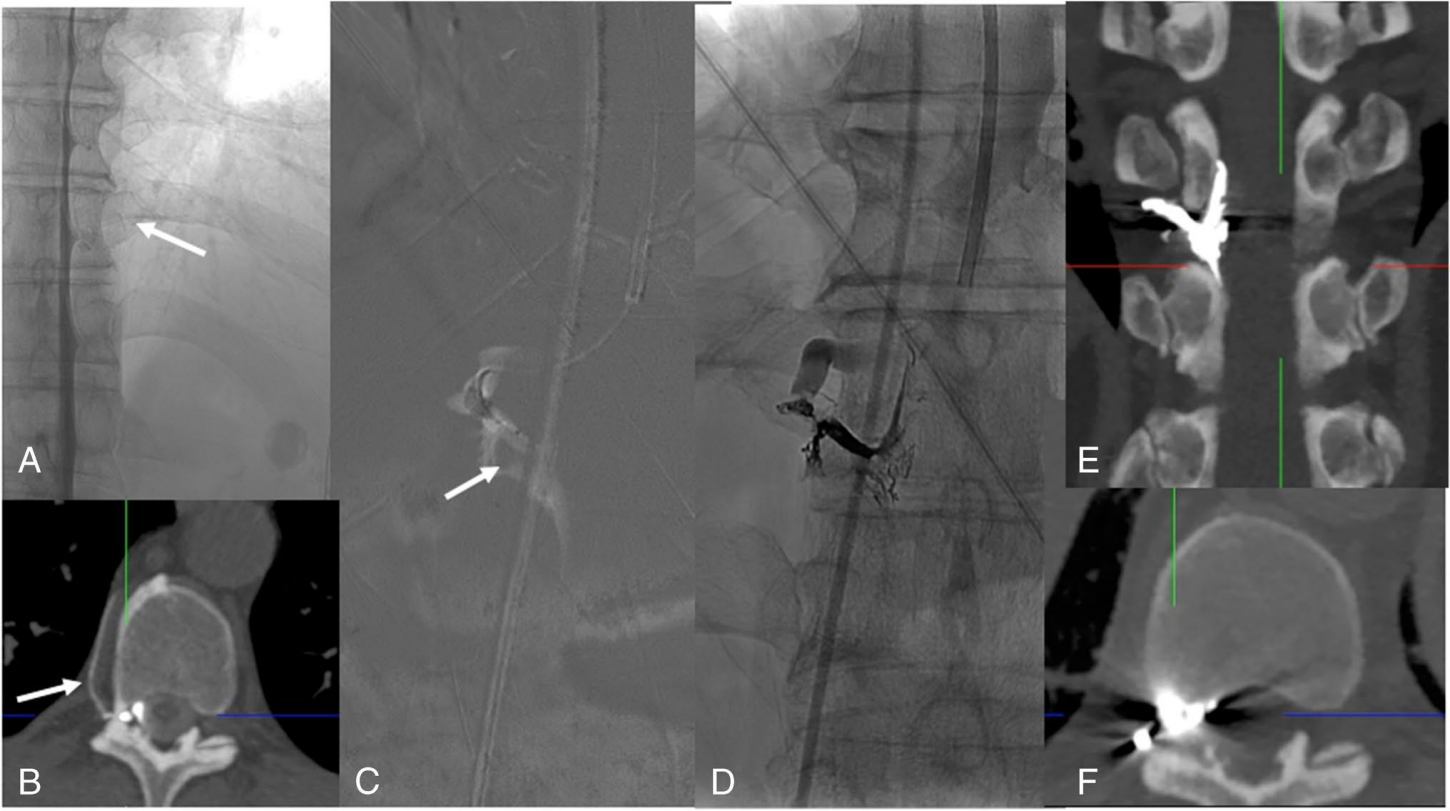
Type 3 leak: Right-sided lateral decubitus dynamic myelography shows a CSF-venous fistula, i.e., here, the tiny venous filling at the level Th 10/11 (A: arrow). Right-sided lateral decubitus CT myelography clearly shows a hyperdense paraspinal vein confirming a CSF-venous fistula (B: arrow). For transvenous embolization, a Scepter dual‐lumen balloon catheter (Microvention, Tustin, CA, USA) is navigated via the azygos vein into the collector of the veins surrounding the nerve root (C: The position of the nerve root is marked with an arrow). Onyx deposition (D). Post-embolization CT shows Onyx cast in the desired position (E, F)
